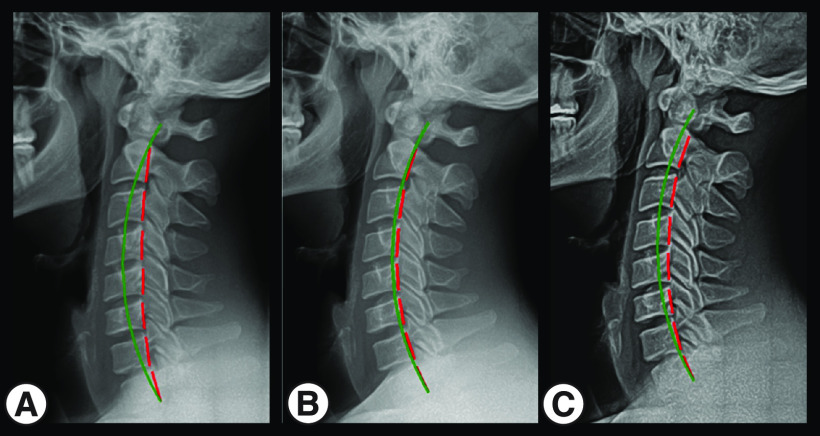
(A) Pretreatment, (B) post-treatment and (C) 2-year follow-up neutral lateral cervical radiographs.
